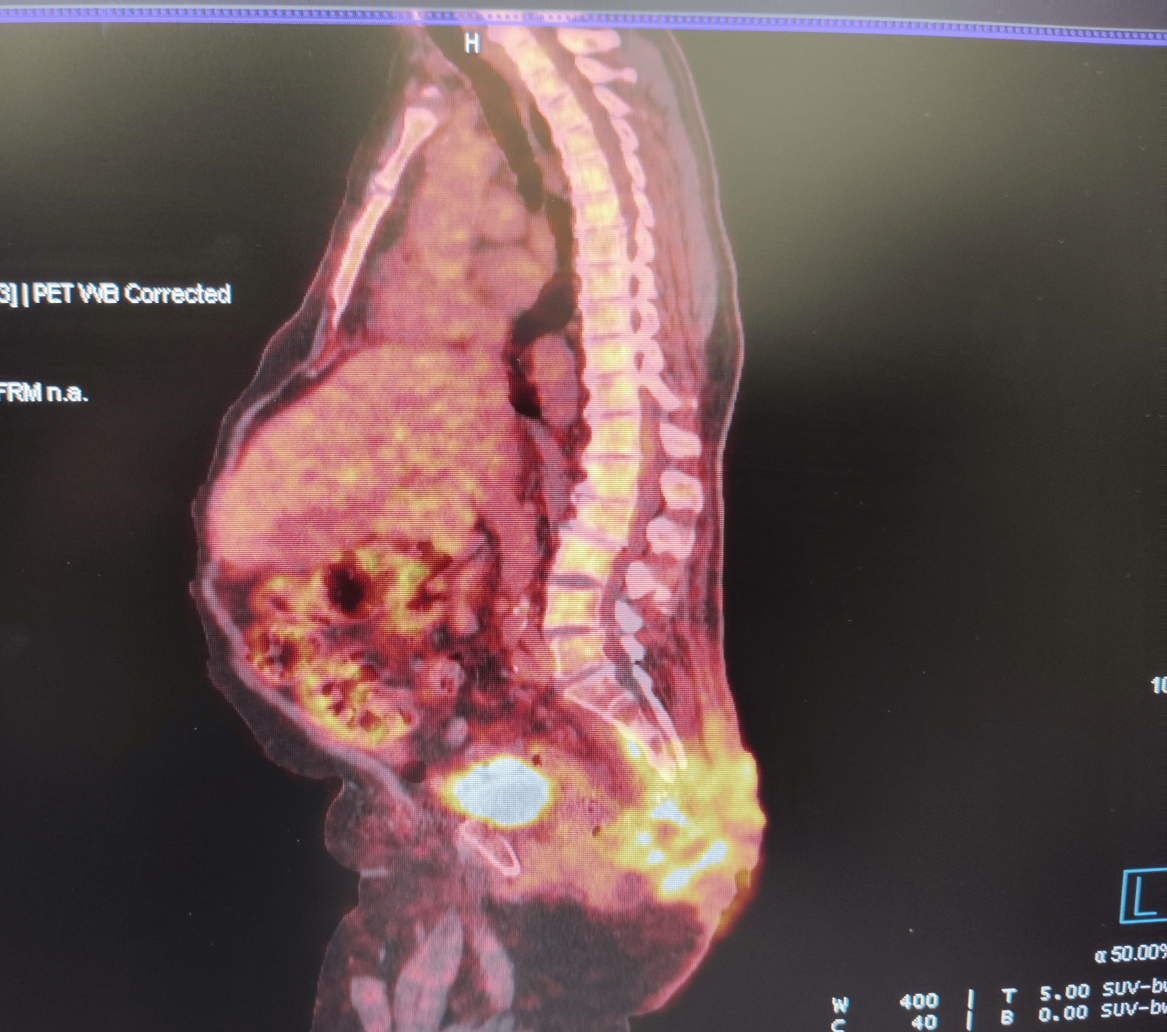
PET/CT performed in May 2024, three months after treatment initiation, showing significant reduction in metabolic activity and partial tumor regression in the sacral and gluteal areas.
